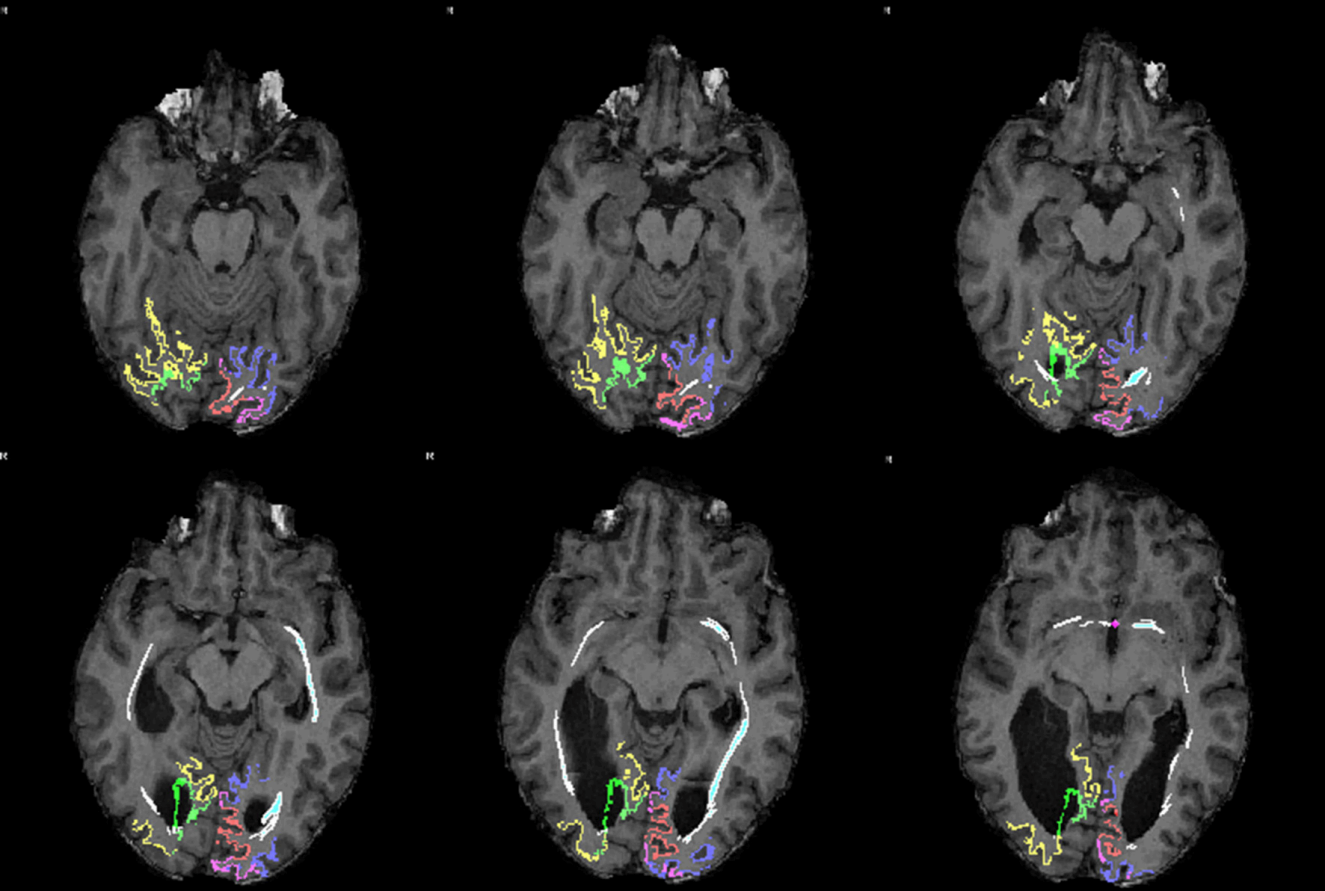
Sequential mid-horizontal axial MRI/DTI sections showing V1 (green/pink), V2 (yellow/purple) and a (white) DTI tract connecting the primary cortical visual areas bilaterally via the anterior commissure in the individual without corpus callosum. Seeds were placed in the primary visual areas with the anterior commissure as region of interest. It shows the fiber tract association with the primary visual areas on both sides.
